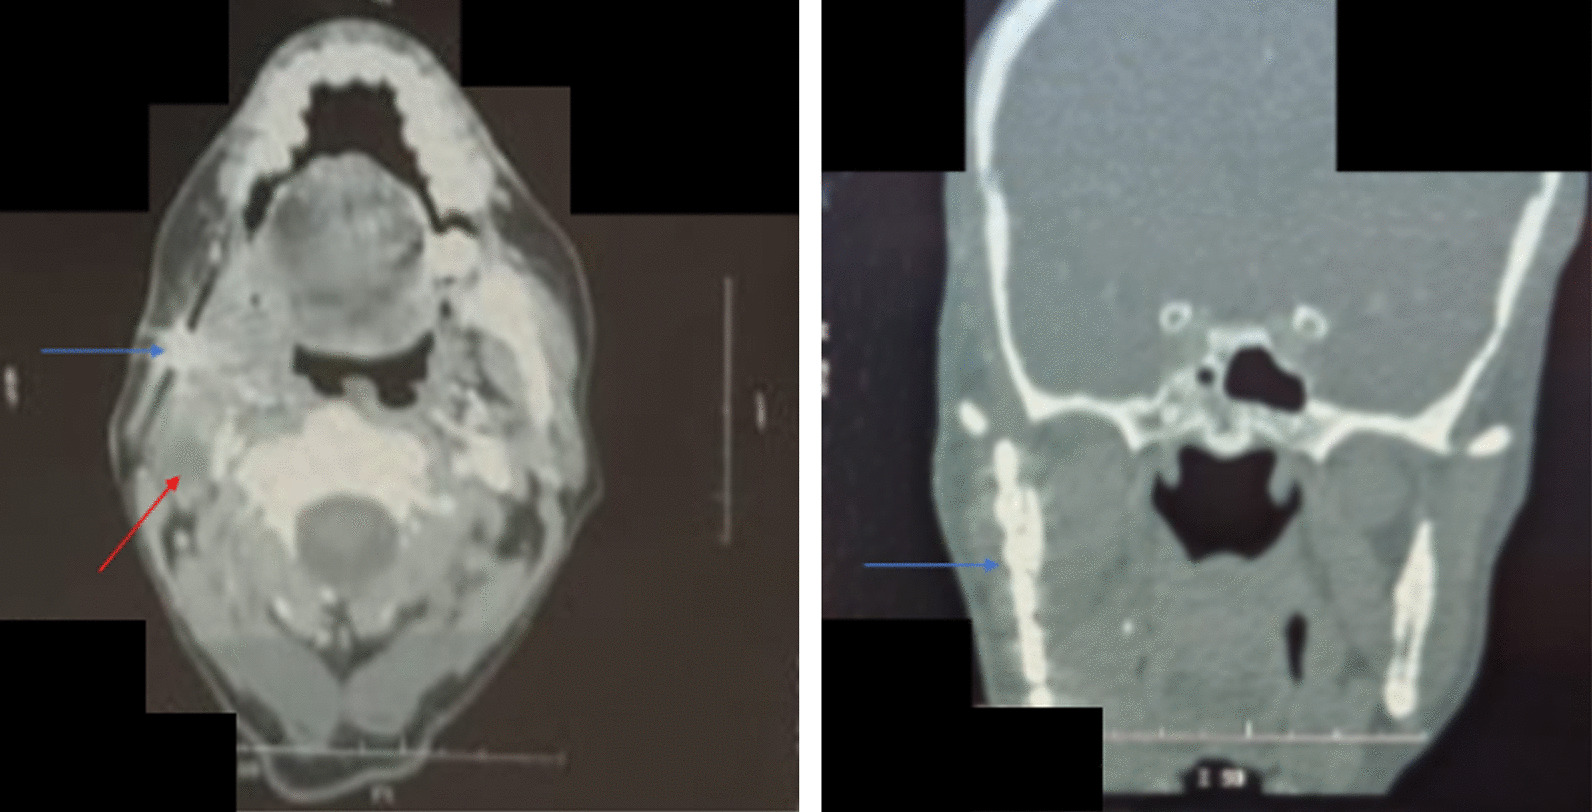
CT scan showing jugulocarotid lymphadenopathy measuring 33 × 15 mm with a necrotic center (red arrow) and angled reconstruction plate (blue arrow)
